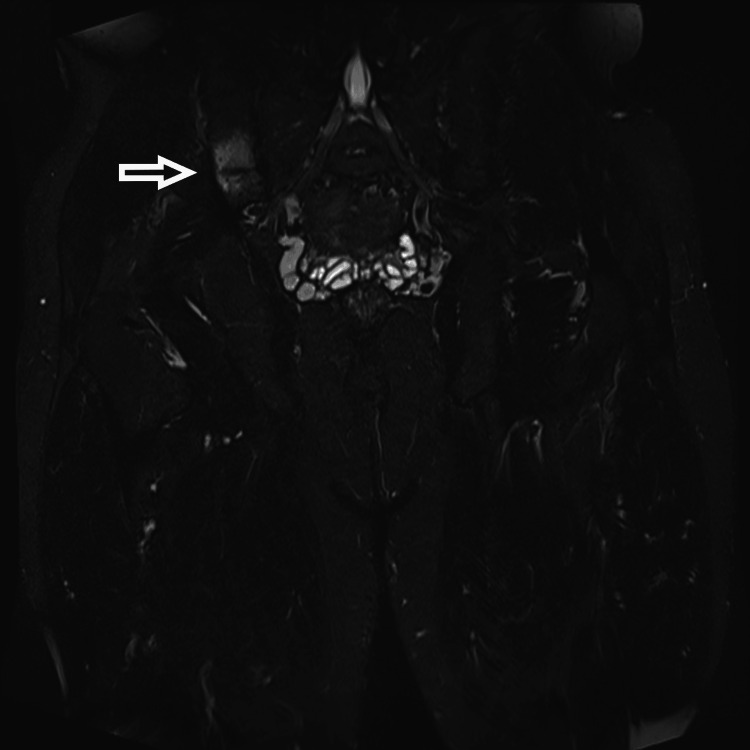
Initial MRI with coronal view STIR showed a partial fracture of the right ilium with bone marrow oedema (arrow) STIR - Short Tau Inversion Recovery
